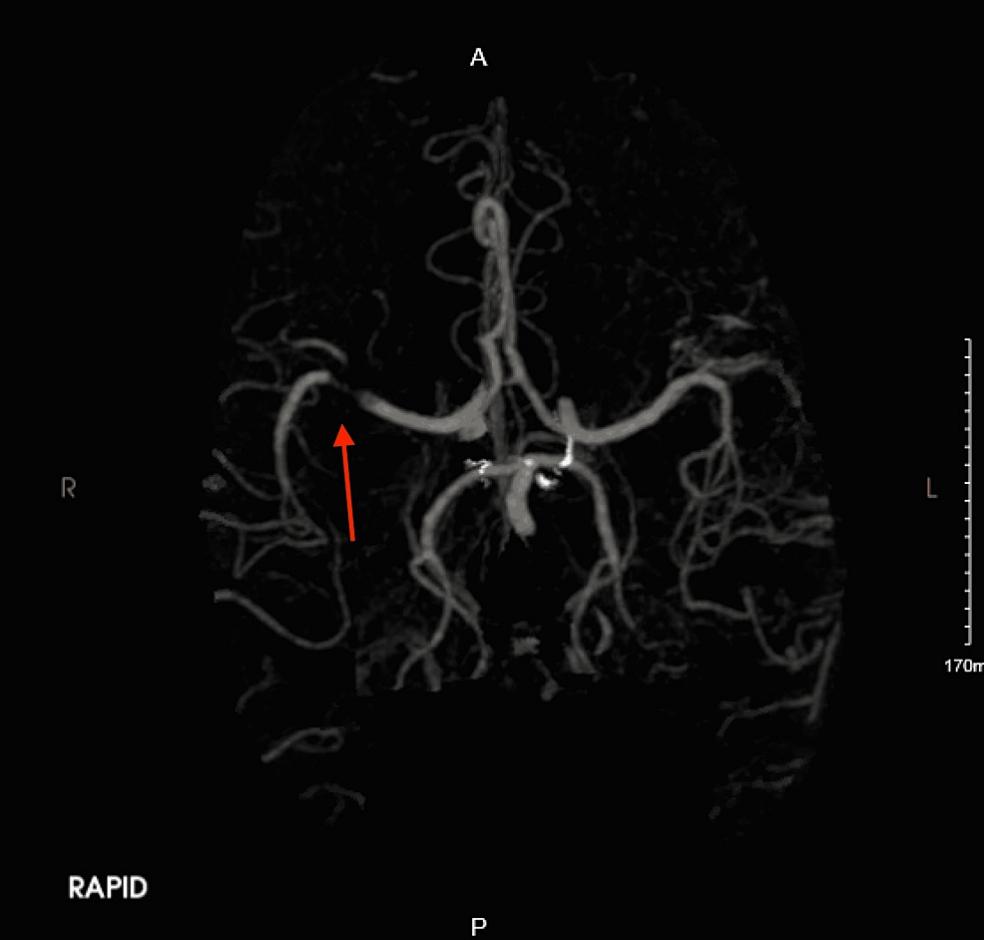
Head CTA revealed a 14 mL perfusion defect involving the left posterior frontal cortex (arrow)
